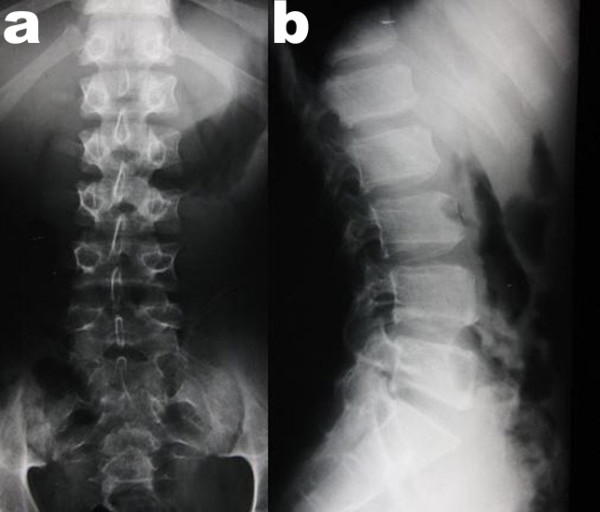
X-rays of the lumbar spine (a: anterior posterior and b: lateral view) demonstrating mild lumbar scoliosis and defects of the anterior cortical rims of the vertebral endplates.
